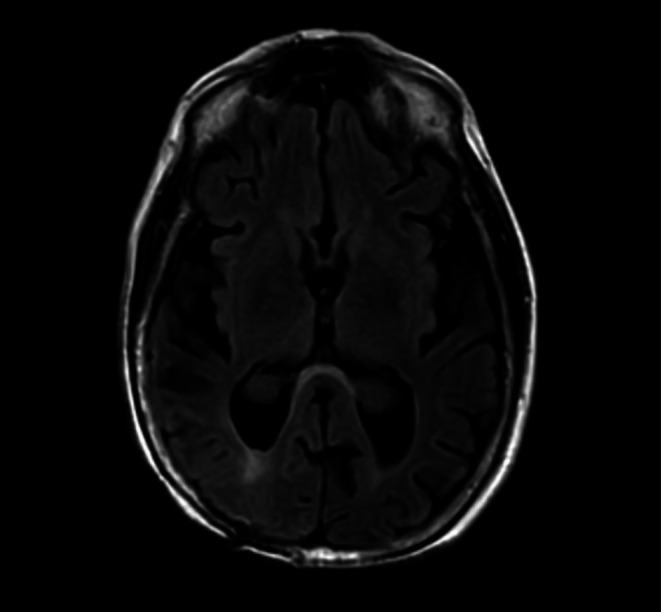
MRI brain showed a right parietal cranioplasty with a post‐surgical cavity in the paramedian right parietal lobe, gliosis, and benign choroid plexus xanthogranuloma.
